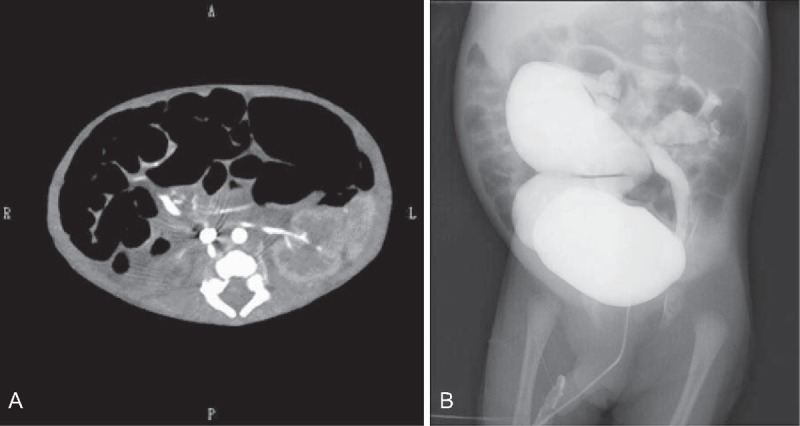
(A) CT image acquired at the level of the bilateral kidney. The right kidney of the patient was small compared with the left kidney. (B) VCUG revealing the VUR and dilation of the right renal pelvis and ureter. CT = computed tomography, VCUG = voiding cystourethrography, VUR = vesicoureteral reflux.
